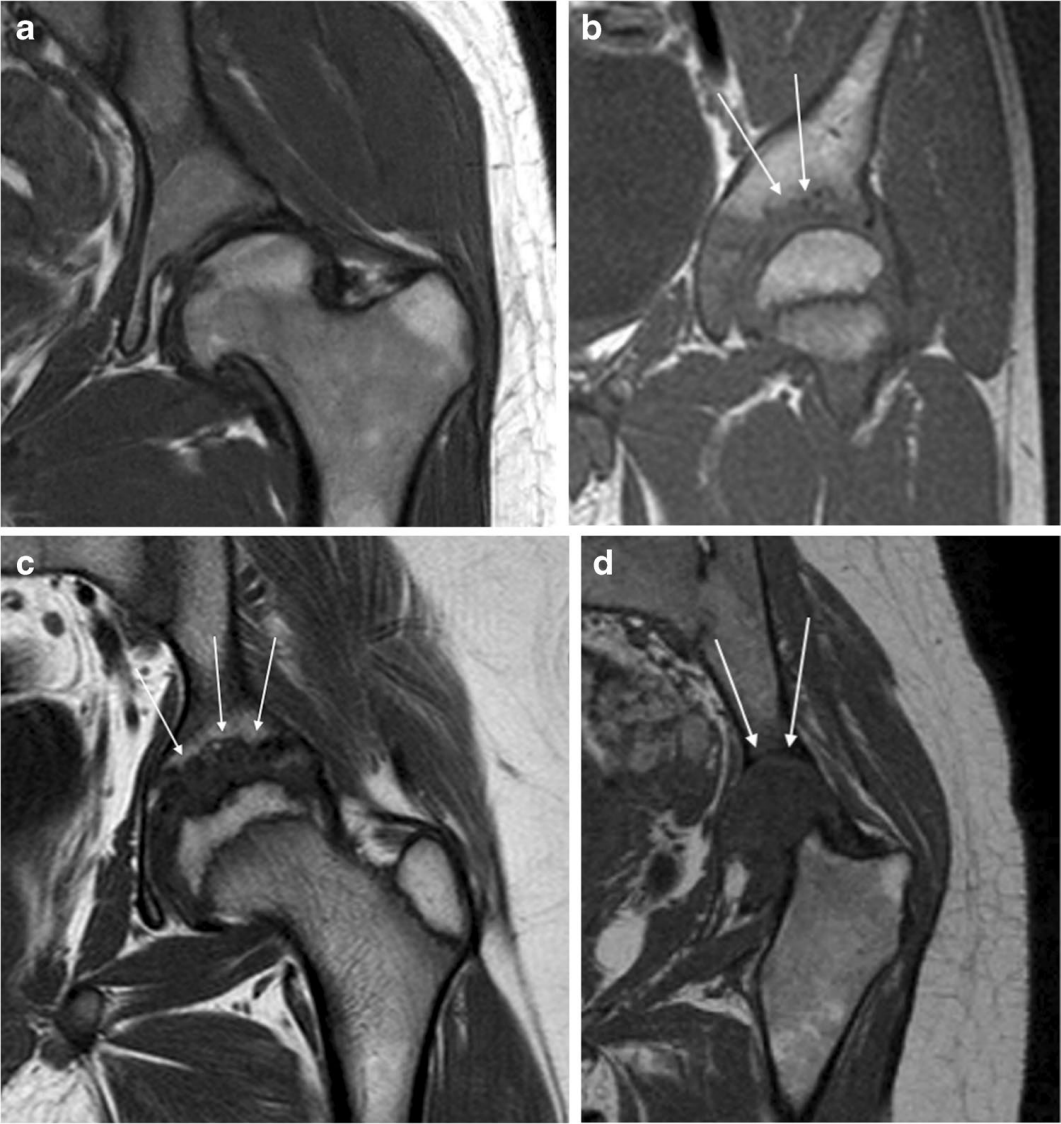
Erosions demonstrated on MRI at the left acetabulum as shown on coronal three-dimensional (3-D) T1-weighted turbo spin-echo sequences in patients with juvenile idiopathic arthritis (JIA). a No visible acetabular erosions (score 0) in a 20-year-old woman. b Some erosions on the superior aspect of the acetabulum (< 33% of the surface, *arrows*) (score 1) in a 18-year-old man. c Multiple acetabular erosions (between 34% and 66% of the surface, *arrows*) (score 2) in a 13-year-old boy. d Erosive changes of the whole acetabular surface (*arrows*) (score 3) in a 19-year-old woman with complete destruction of the femoral head (*arrows*)
